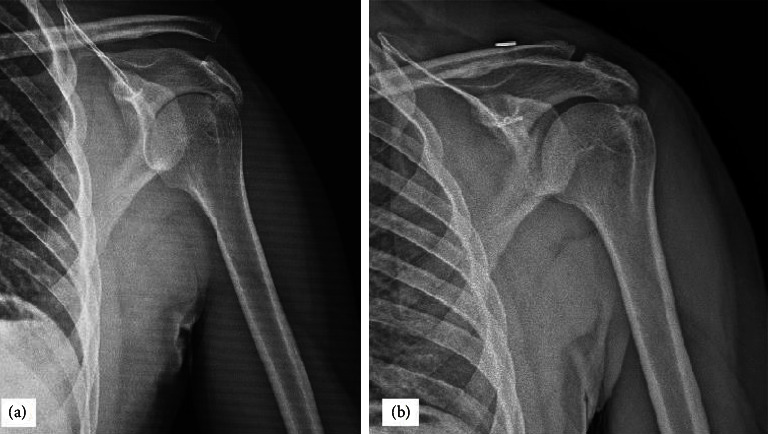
(a) The preoperative anterior and posterior view of a 42-year male patient, who had Rockwood type III acromioclavicular joint dislocation at his left shoulder due to the fall from standing height. (b) 6-month postoperative plain radiograph showing good maintenance of joint reduction with minimally invasive TightRope.
